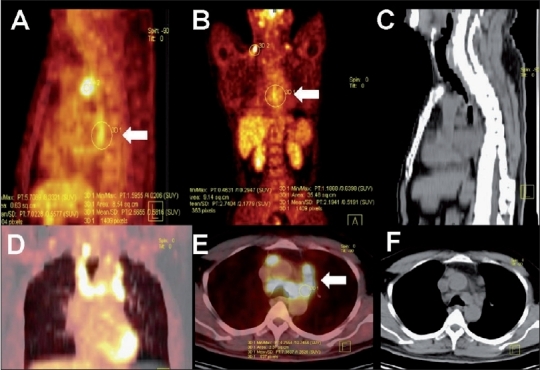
Tuberculosis of the esophagus. Endoscopy in a 42-year-old man with dysphagia revealed a mid-esophageal stricture. Sagittal (A) and coronal (B) PET, sagittal plain CT (C), coronal PET/CT (D), axial PET/CT (E), and axial CT (F) images of the thorax show increased FDG uptake in the mildly thickened walls of the mid-esophagus (arrows), with multiple FDG-avid nodes in the mediastinum and supraclavicular regions. Carcinoma of the esophagus was suspected. Biopsy from the esophagus and the supraclavicular lymph nodes revealed tuberculosis
